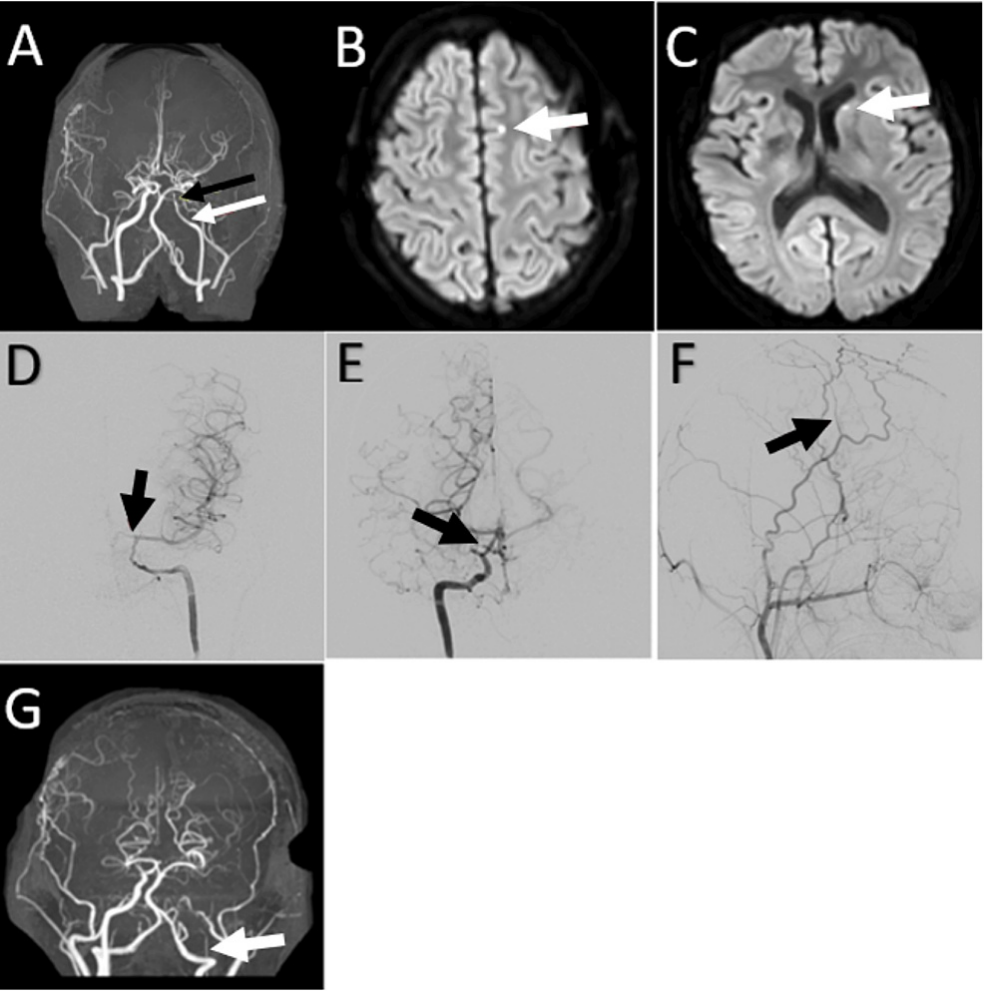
Cranial imaging revealing progressive moyamoya arteriopathy of left-sided vasculature 3a: Coronal MRA MIP from February 2021 showing increased stenosis of the left ICA beginning in the petrous segment (white arrow) and progressing distally (black arrow). 3b: Axial MRI diffusion-weighted images (DWI) obtained three months after left EDAS showing hyperintensity (arrow) in the left superior frontal gyrus consistent with acute ischemia. 3c: Axial DWI MRI obtained three months after left EDAS showing hyperintensity (arrow) in the left caudate head consistent with acute ischemia. 3d: AP left ICA angiogram showing severe left anterior cerebral artery stenosis (arrow). 3e: AP right ICA angiogram showing severe right ACA stenosis (arrow) not seen on previous angiograms (Figure 2e). 3f: Lateral left external carotid artery angiogram showing a patent superficial temporal donor artery (arrow) used for EDAS with no apparent collateral ingrowth to the brain. 3g: Coronal MIP from the most recent MRA showing severe progression of left ICA stenosis into the cervical segment (arrow).
